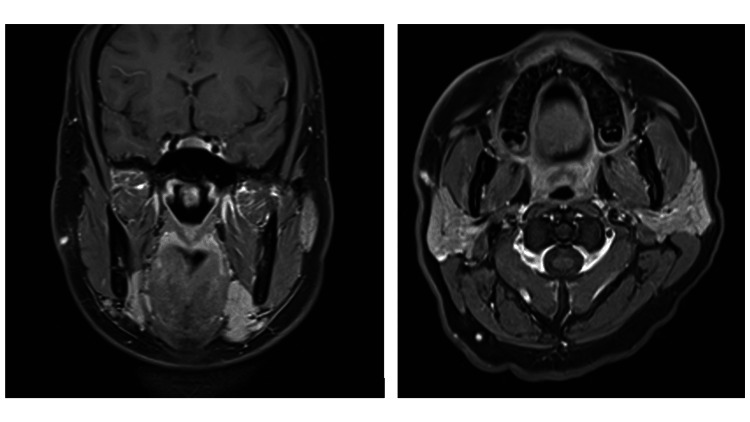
MRI imaging demonstrating seeding through the tract following core biopsy Left image: coronal view; Right image: axial view
